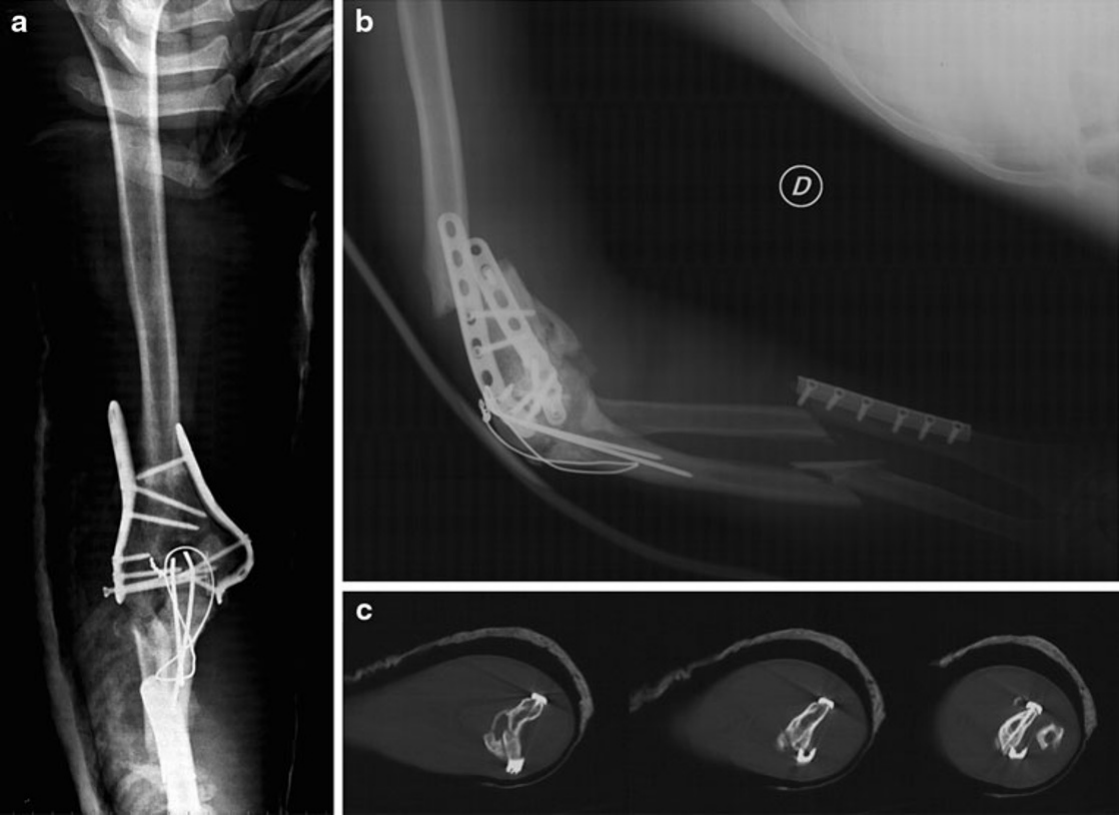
AP (a) and lateral (b) radiographs at hospital admittance show supracondylar fracture of the humerus and midshaft fractures of radius and ulna (second floating elbow injury). The CT scan (c) of distal humerus demonstrates the intercondylar integrity with an intra-articular bridge resulting from the previous treatment
